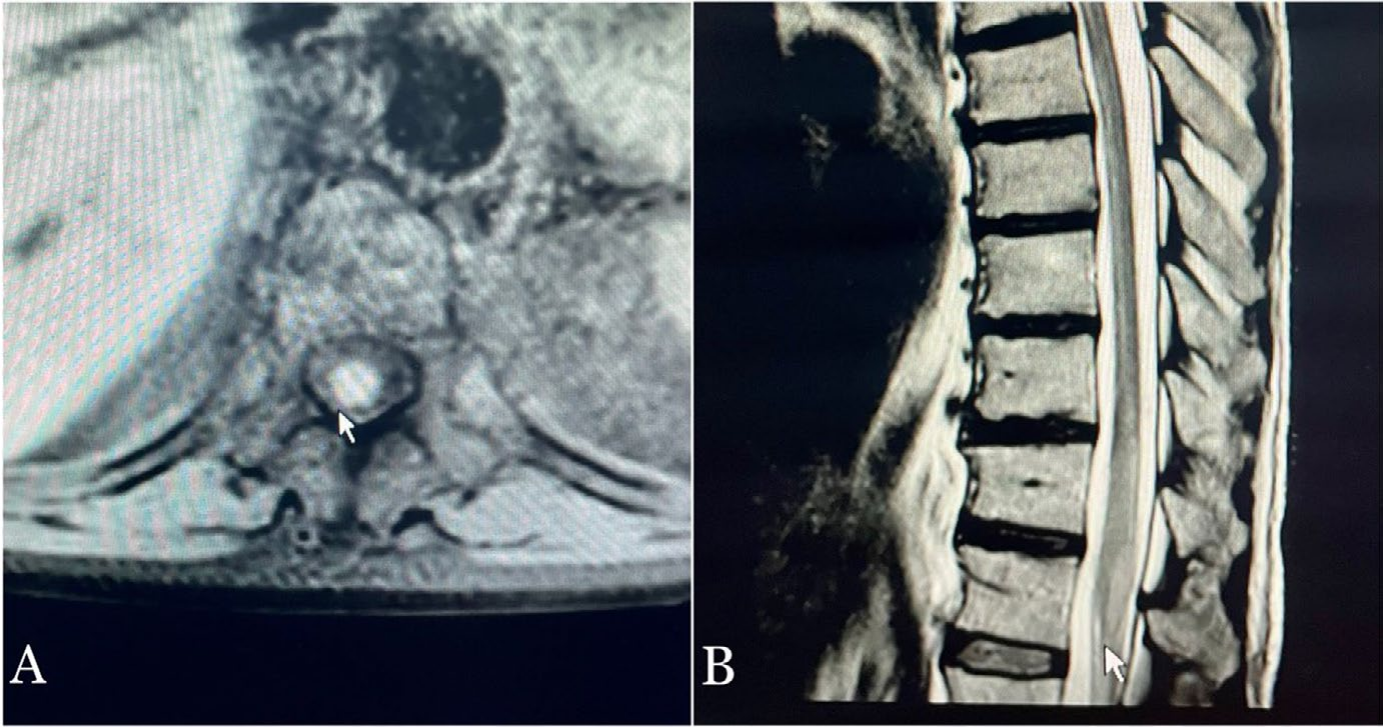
MRI of the entire spine with gadolinium contrast. (A) Axial T2‐weighted image at the level of T11 demonstrating a hyperintense signal within the spinal cord, suggestive of inflammation and edema. (B) Sagittal T2‐weighted image shows hyperintense signal changes at the level of T10–T12 thoracic vertebrae, indicative of an inflammatory process without compressive lesions. These findings are consistent with transverse myelitis.
